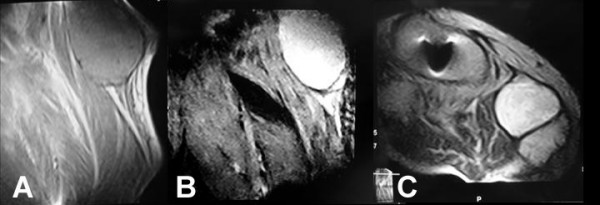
(A) A sagittal T1-weighted magnetic resonance image of the right knee shows a low signal lesion with a maximal diameter of 8 cm at the origin of the medial head of the gastrocnemius muscle. (B) A sagittal T2-weighted magnetic resonance image shows a high signal fluid-filled lesion with inner septums and thin outer wall. (C) A transverse T2-weighted magnetic resonance image shows a cystic fluid-filled lesion at the postero-medial aspect of the knee joint.
